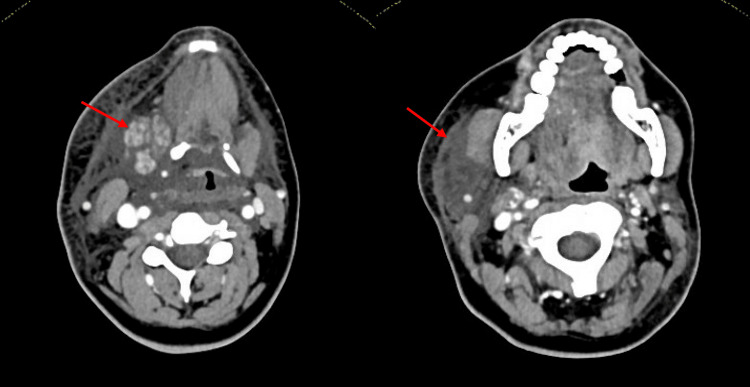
Post-operative CT of the neck region shows a remarkable swelling of the submandibular gland with patchy contrast-enhancing and infiltrations of the subcutaneous tissues.
